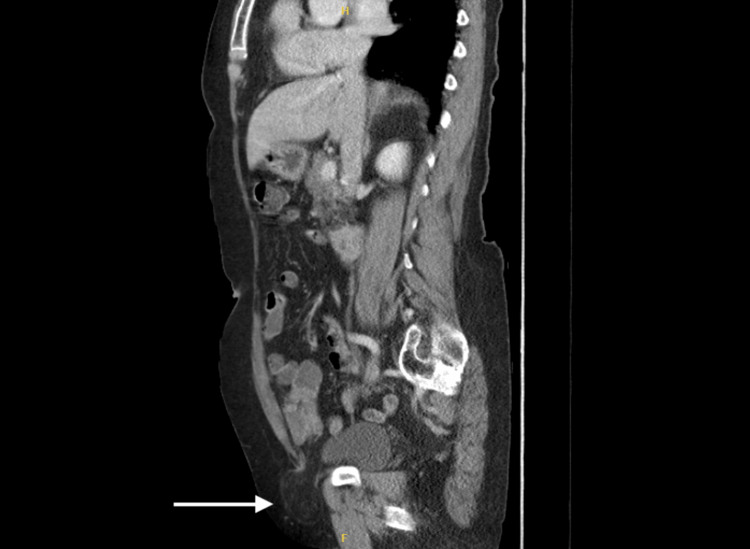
A computed tomography scan demonstrating a right-sided hernia (white arrow).
